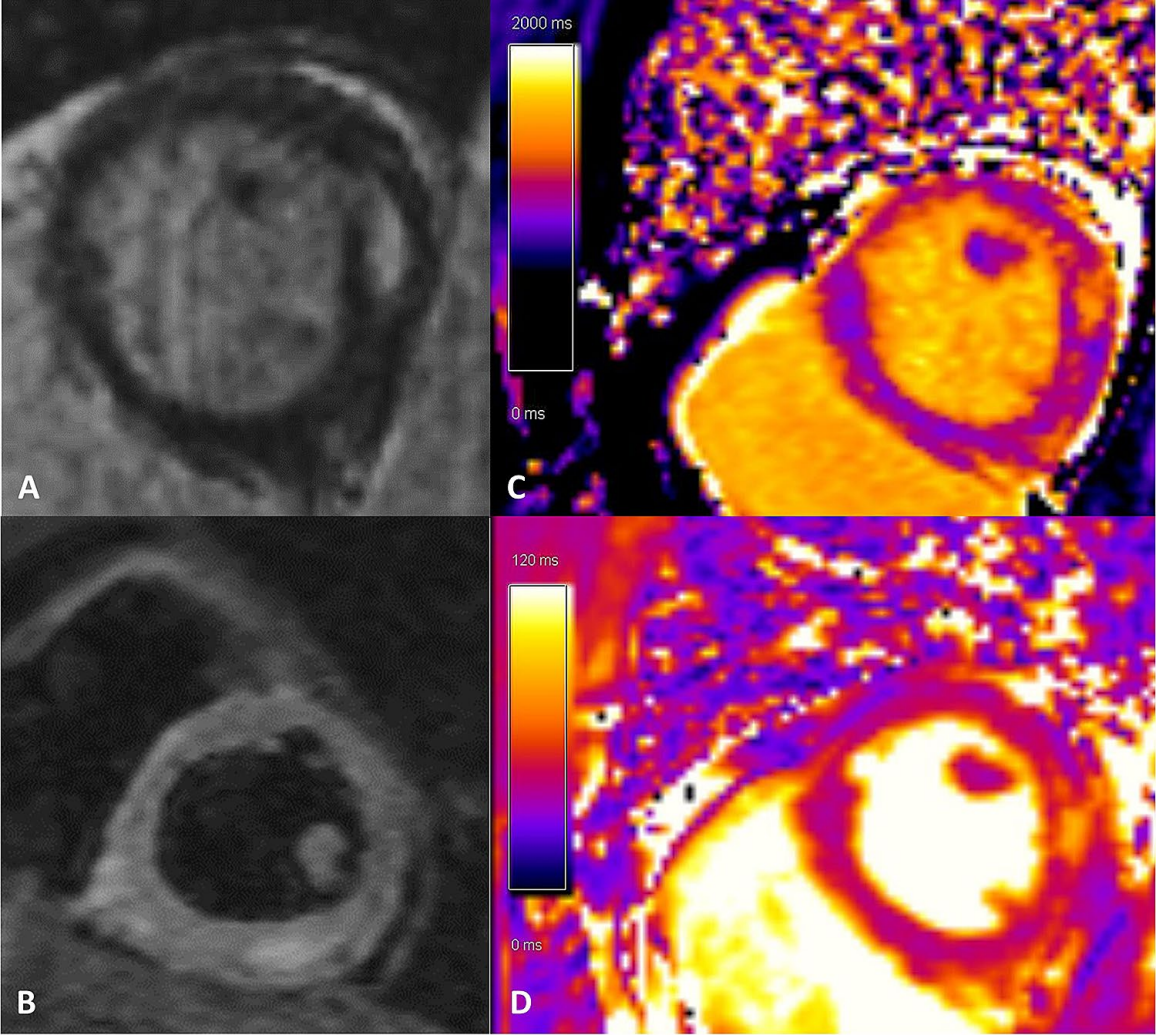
MRI diagnosis of an acute myocarditis. MRI-findings of the patient who fulfilled both Lake Louise criteria with abnormal findings with emphasis basal inferolateral: non-ischemic myocardial fibrosis proven by intramyocardial late gadolinium enhancement (A) and increased T1 times (C) as well as signs of a myocardial edema with pathological TIRM-Sequence (B) and increased T2 relaxation time (D)
